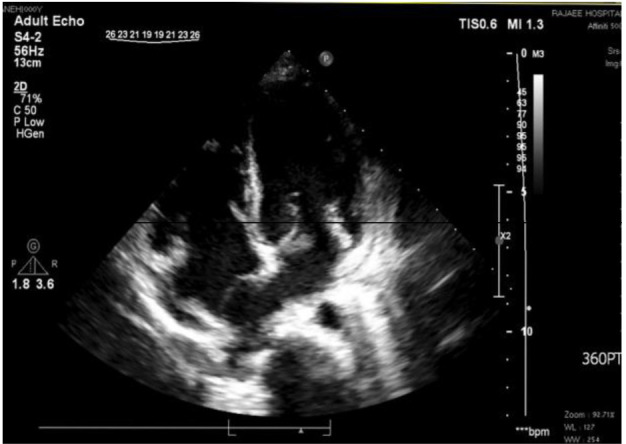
Apical 4 Chamber view showed large fungal vegetation closed to the anterior mitral leaflet hinge point
